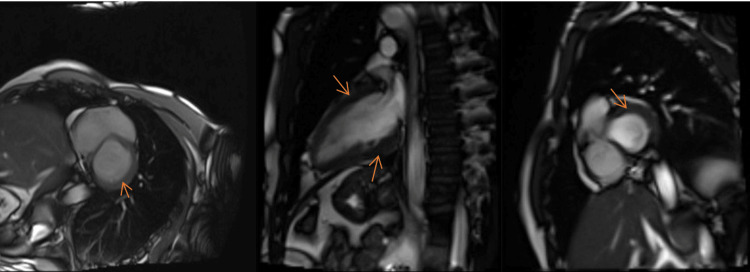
A cardiac MRI demonstrates mildly oedematous mid- to inferior-apical segments with mild hypokinesis and epicardial late gadolinium enhancement, consistent with myopericarditis. Normal left ventricular volume and systolic function are observed, with a left ventricular ejection fraction of 57%.
